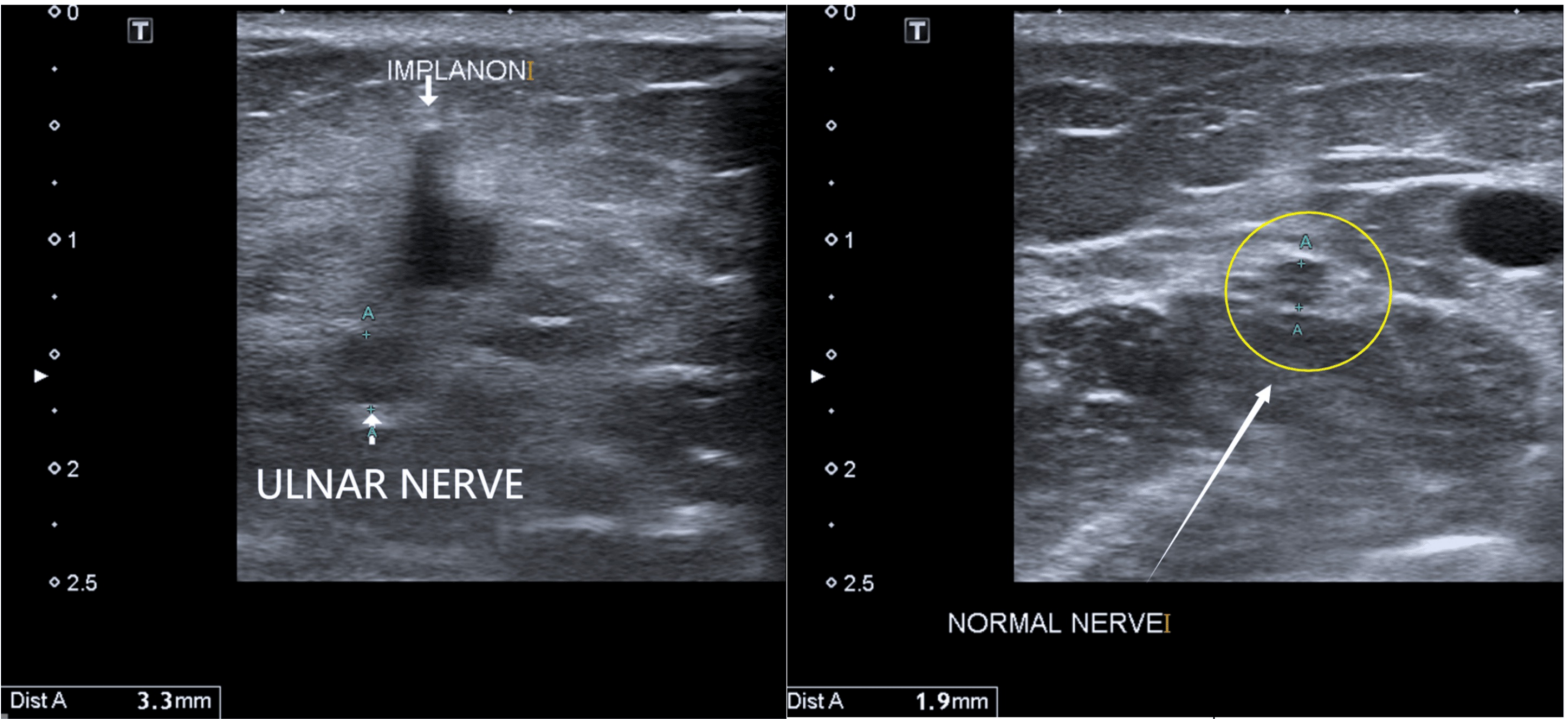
Ultrasound of the left arm showing the ulnar nerve measuring 3 mm compared to the normal 2 mm
